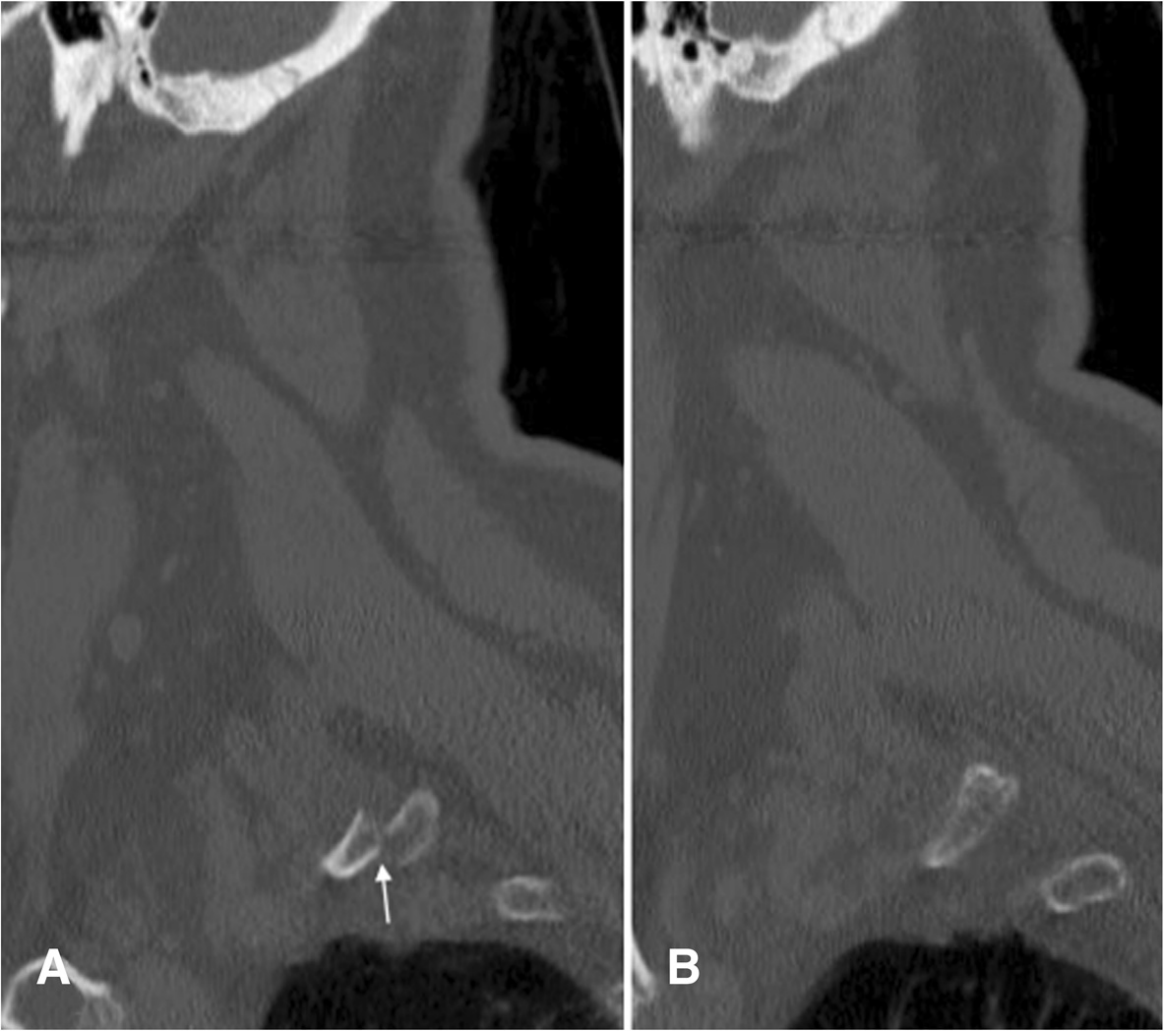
Non-contrast CT of the cervical spine. Sagittal image (A) reveals an anteriorly displaced fracture of the right first rib (white arrow). For comparison, image (B) reveals a normal left first rib.
